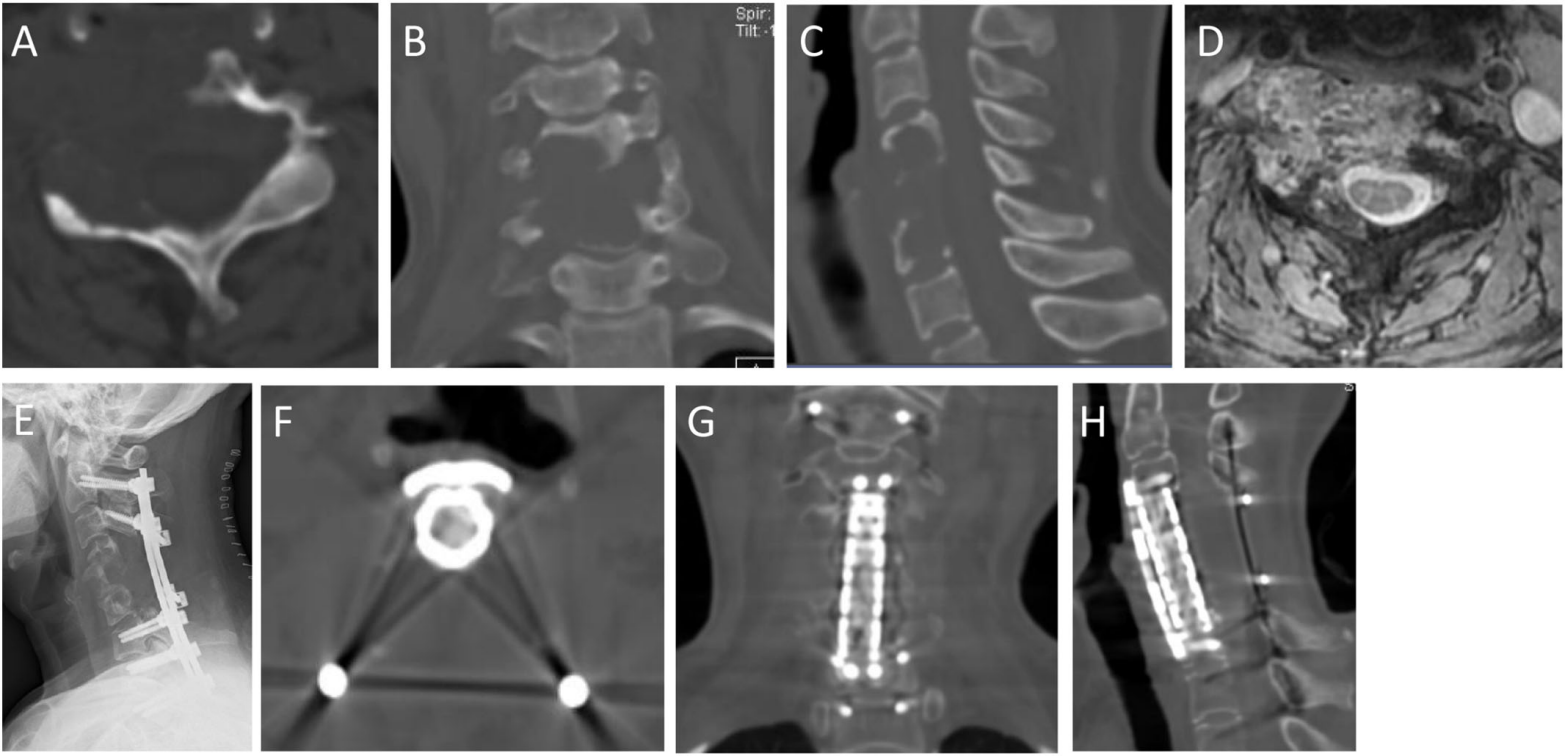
A 47-year-old female with neck pain for 12 months, accompanied with pain and numbness of left upper extremity for the recent 1 month. Preoperative imaging examination showed an osteolytic lesion was in the C4-6. She underwent GTR (piecemeal spondylectomy). At 62-month follow-up, she was symptom free. (A,B,C): Preoperative axial, coronal and sagittal CT scans; (D): T2-weighted axial MRI scans; (E): Postoperative X-ray; (F–H): Axial, coronary and sagittal CT scans in 62th month after surgery.
